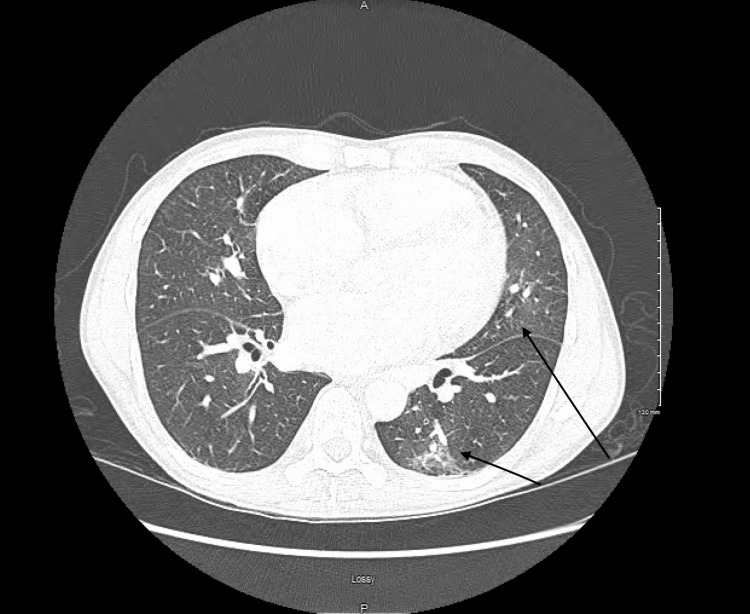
CTPA of the chest showing no signs of pulmonary embolism, patchy ground glass opacities within the lingula and left lower lobe, mild dependent changes with subsegmental atelectasis within the bilateral lower lobes, and mildly enlarged main pulmonary artery (black arrows) CTPA: computed tomography pulmonary angiogram
